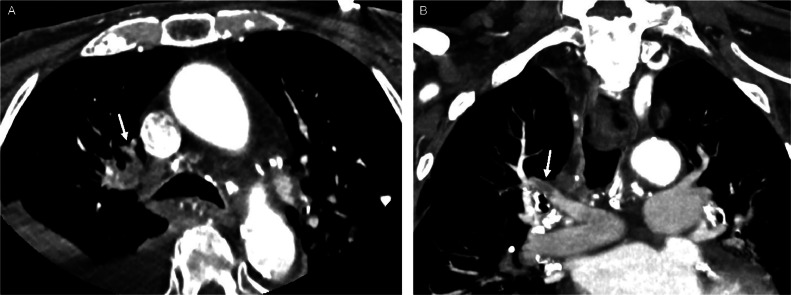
CTA stroke protocol Axial (A) and Coronal (B) images demonstrating pulmonary embolism in right upper lobe pulmonary arteries (arrow).
